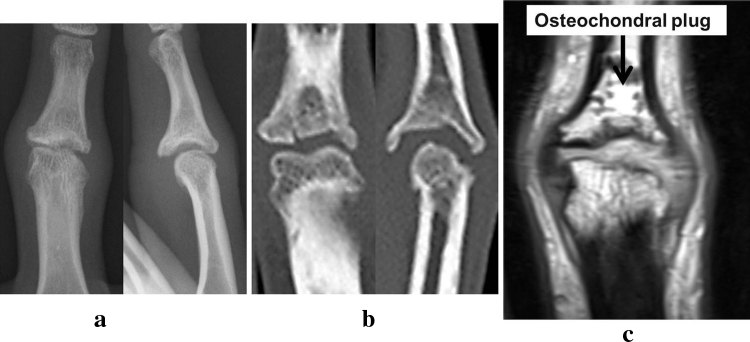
Imaging findings at 1 year after treatment. a, b X-ray and CT images show bone union with no dislocation of the osteochondral plug. Although slight displacement of the finger to ulnar side remained, the ulnar displacement of the axis improved from preoperative 14° to postoperative 8°. c MR image shows well-maintained joint space by the cartilage
